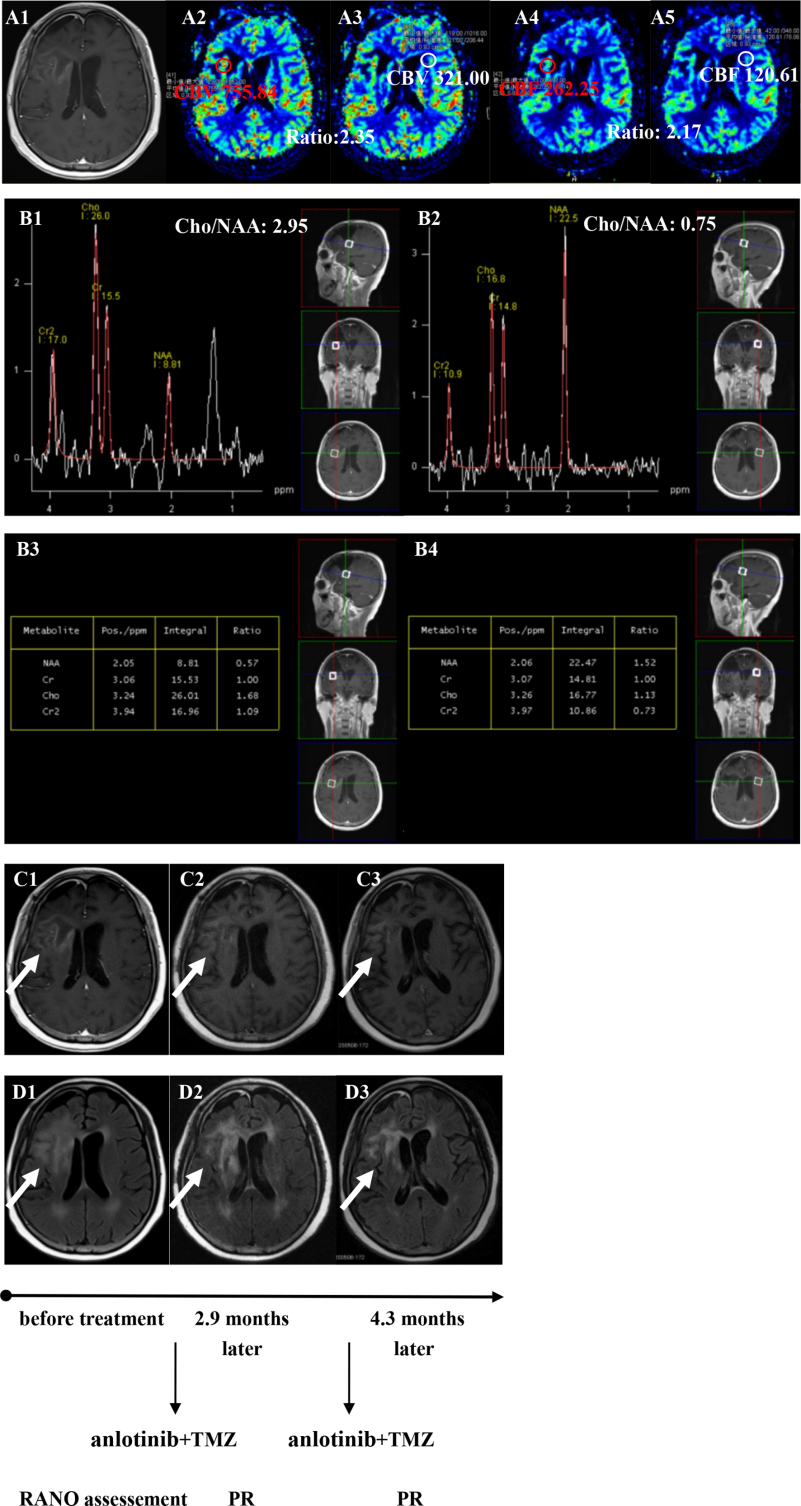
Brain scan of patient number 3 (IDH1−; in situ recurrence. The interval time between the end of CCRT and the treatment of anlotinib combined with TMZ was 3.2 months). (A1–A5) Perfusions maps at the time of recurrence; (B1−B4) MRS at the time of recurrence; (C1–C3) Contrast-enhanced MRI; (D1–D3) MRI-Flair; MRI images followed-up every 1 to 3 months before and after treatment. The arrow refers to the area of recurrence. The patient achieved partial remission after treatment and had a progression-free survival time of 5.8 months.
